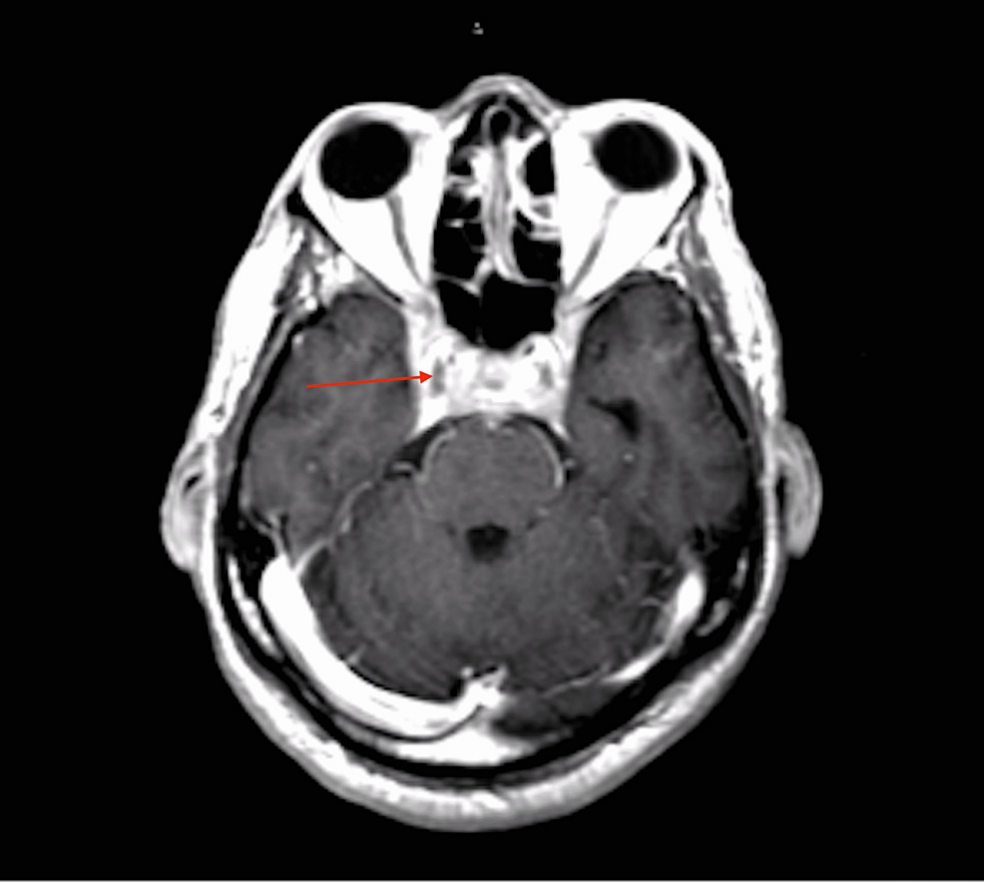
Post-contrast T1 MRI Brain Demonstrating Diffuse Abnormal Thickening and Enhancement of the Orbital Apices, Superior Orbital Fissures, Cavernous Sinuses, and Sella
